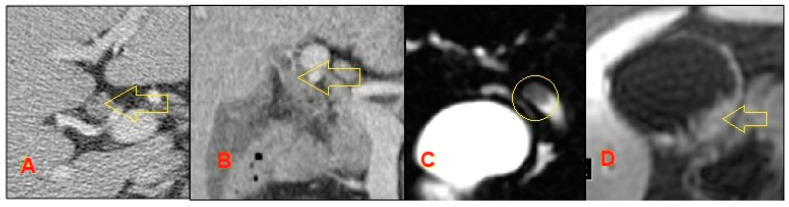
A,B: Axial contrast computed tomography (CT) shows enhancing intraductal polypoid mass consistent with intraductal cholangiocarcinoma (arrow); C: The circle demonstrates a T2W hypointense lesion found on magnetic resonance cholangiopancreatography (MRCP); D: Postcontrast MR showing an incidentally found subtle mural thickening of the gallbladder fundus later diagnosed as synchronous gallbladder carcinoma.
